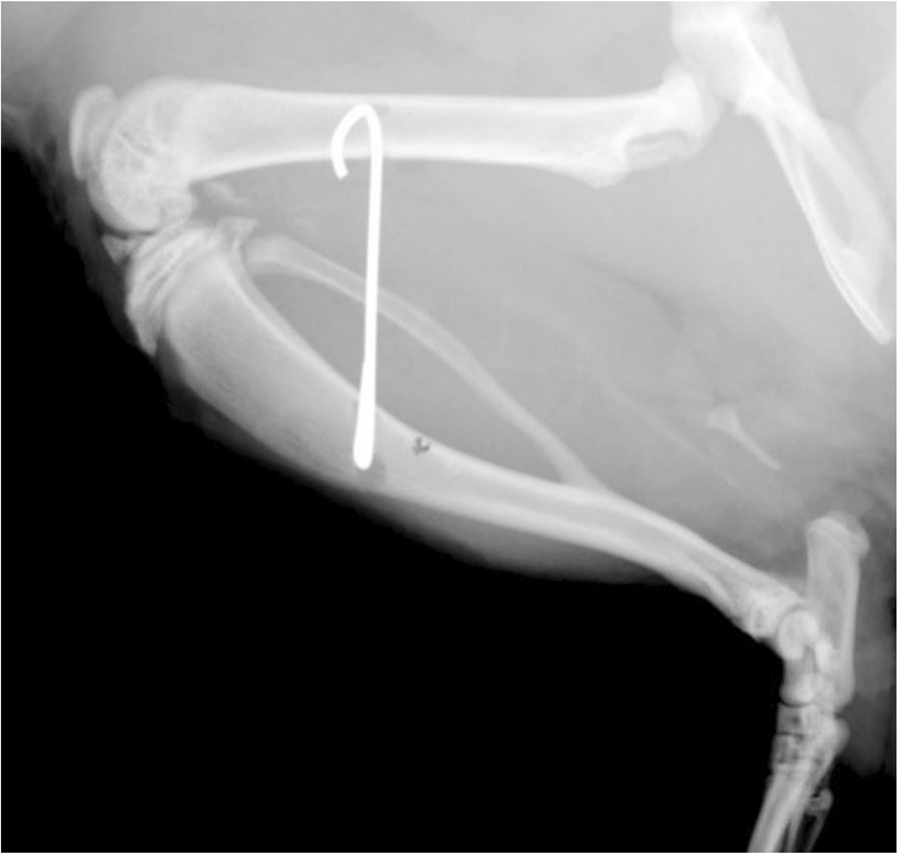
Postoperative lateral X-ray of the knee joint. K-wire immobilization in a 145° flexed knee position
